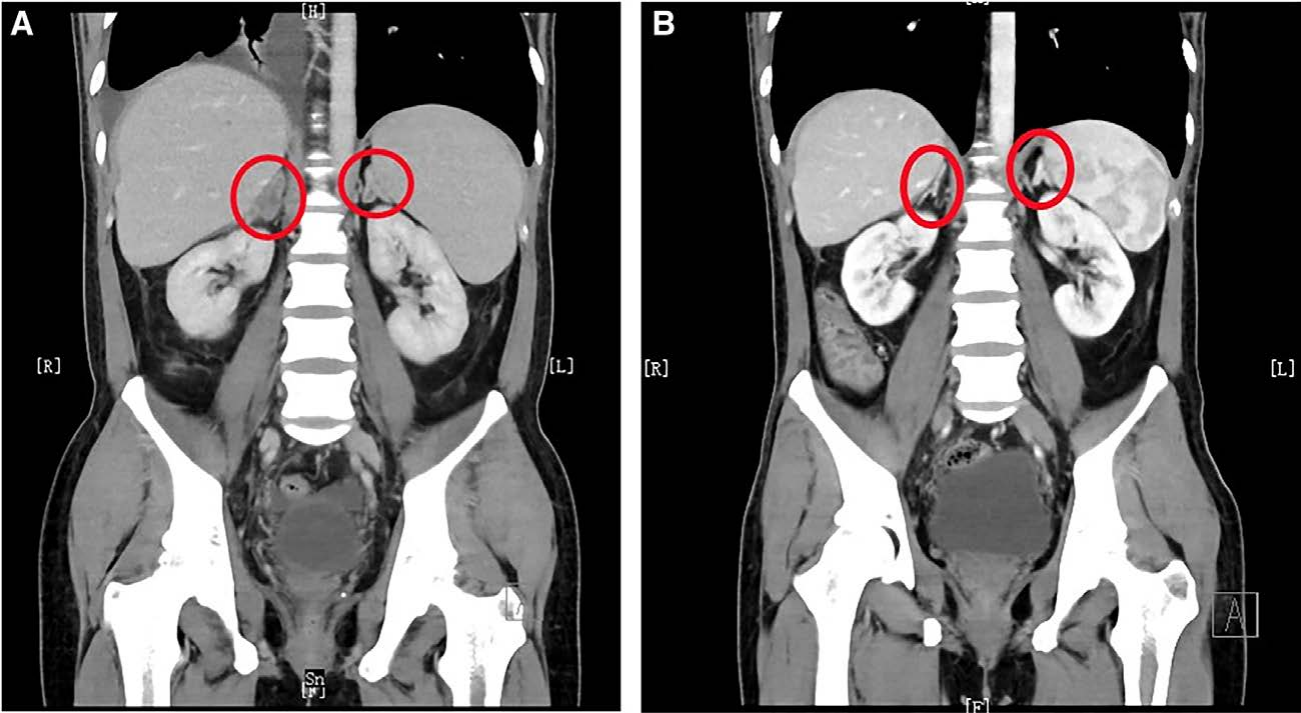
Before treatment, CT showed that bilateral adrenal glands were significantly thickened and decreased in density, and low-density shadows were seen after enhanced scanning (A). After treatment, CT showed that the left adrenal gland was thickened, the enhancement was not uniform after enhanced scanning, and the abnormal enhancement shadow of the original right adrenal gland disappeared (B). Peritoneal and pelvic effusions were reduced.
